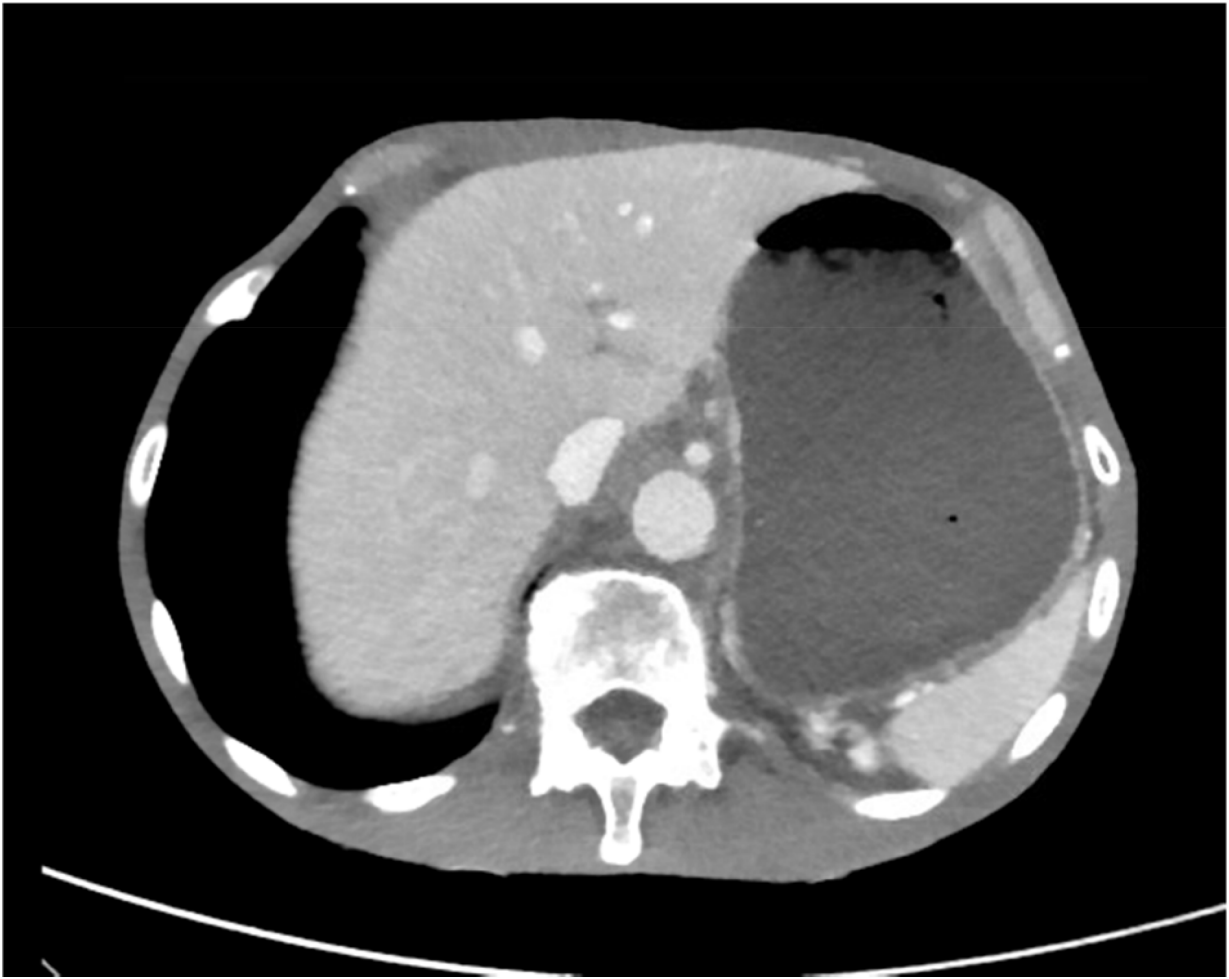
CT imaging of gastric distention.
